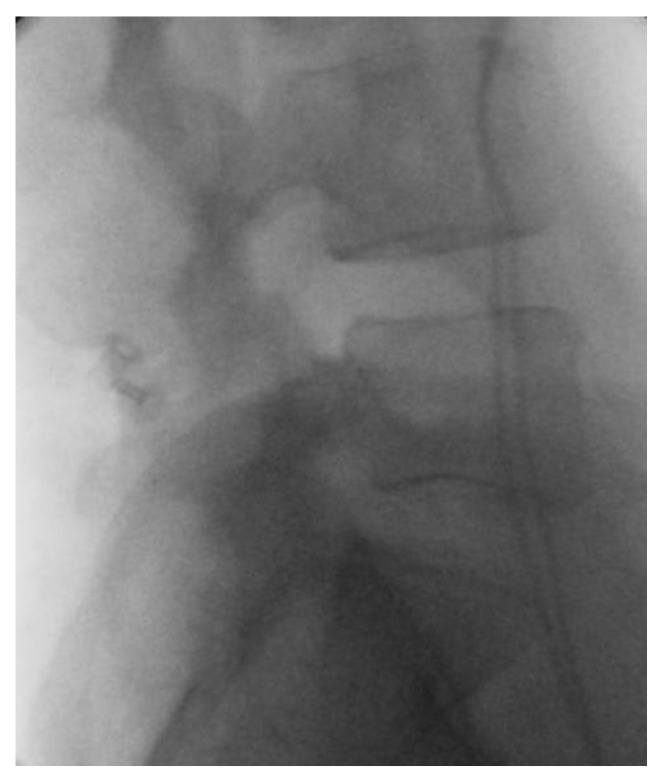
Interspinous spacer. Postoperative X-ray after interspinous spacer implantation (L4–L5 segment).
